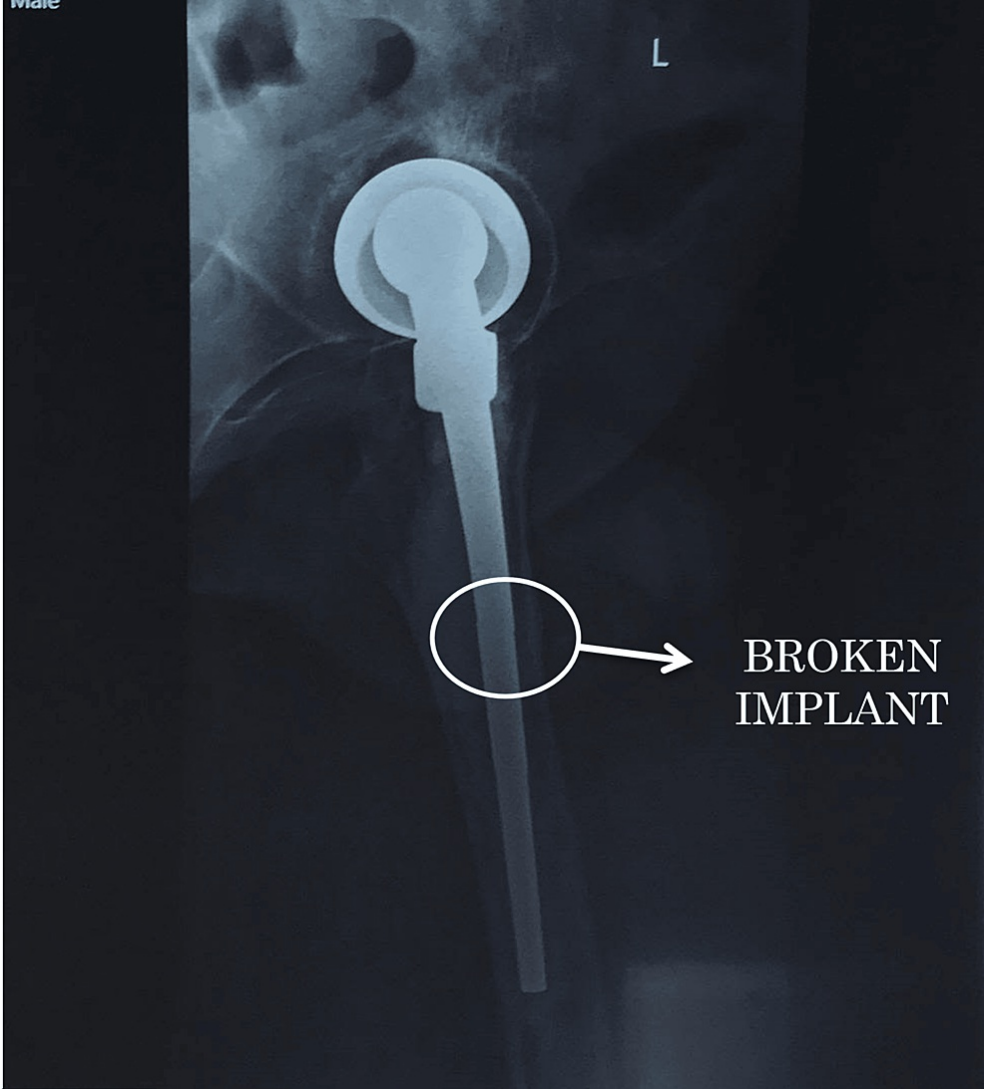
Plain radiograph of the left hip lateral view showing the broken implant
